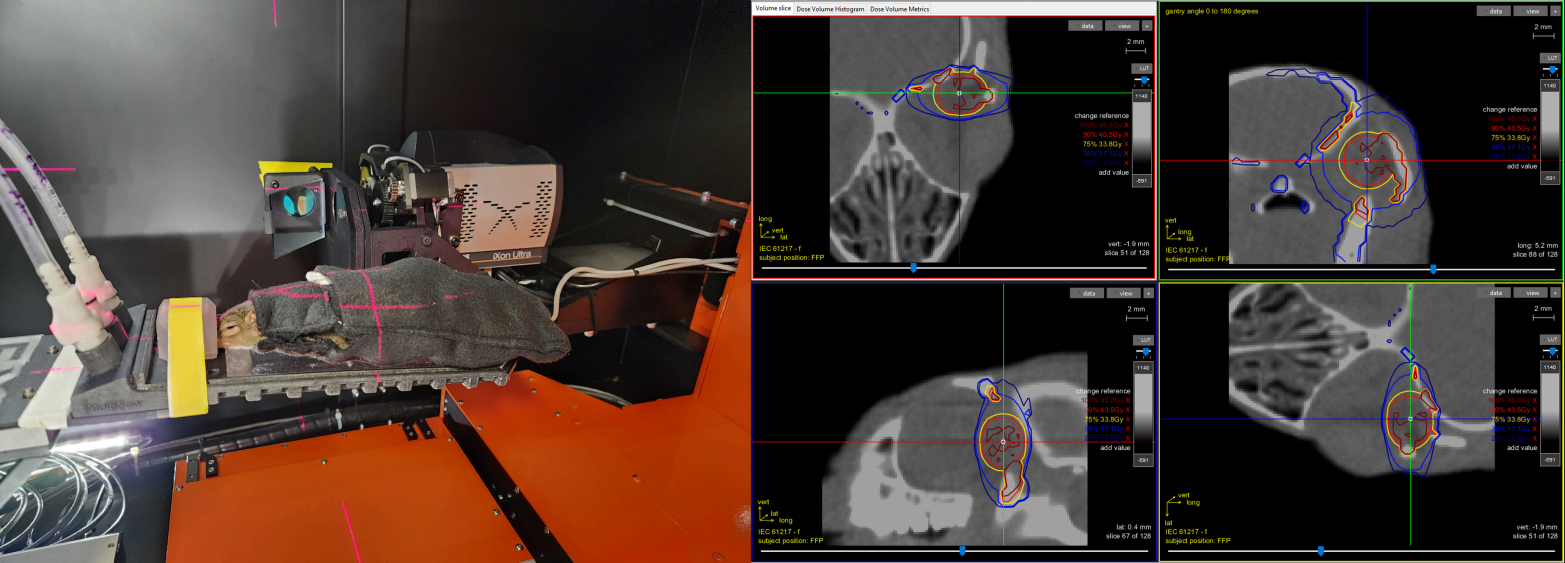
Radiation setup and targeting. Tree shrews were mounted on the stage for radiation procedures (left). Radiation targeting was planned using bony landmarks visualized with real-time CT scans (right), with confirmation by imaging the optic nerve and posterior edge of the globe. The software provides a 4-panel view to assist with radiation targeting.
